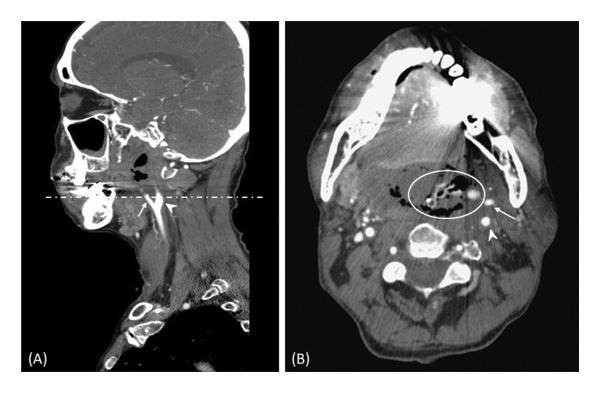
First episode of carotid blowout syndrome. Contrast‐enhanced computed tomography angiography (CTA) in (A) sagittal and (B) axial views reveals extensive necrotic tissue infiltrating the left carotid artery. The circle highlights active contrast extravasation originating from the proximal segment of the left external carotid artery (ECA). Arrows indicate the ECA, and arrowheads indicate the internal carotid artery (ICA). The dashed line in (A) denotes the corresponding axial level (B).
